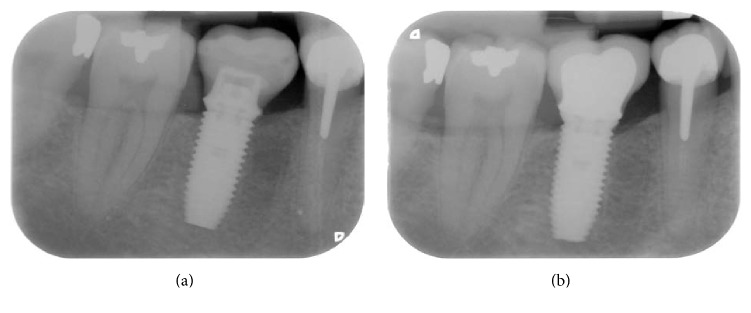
X-ray radiographs of the implant and the restoration (a) at implant insertion and (b) 1 year after surgery.
